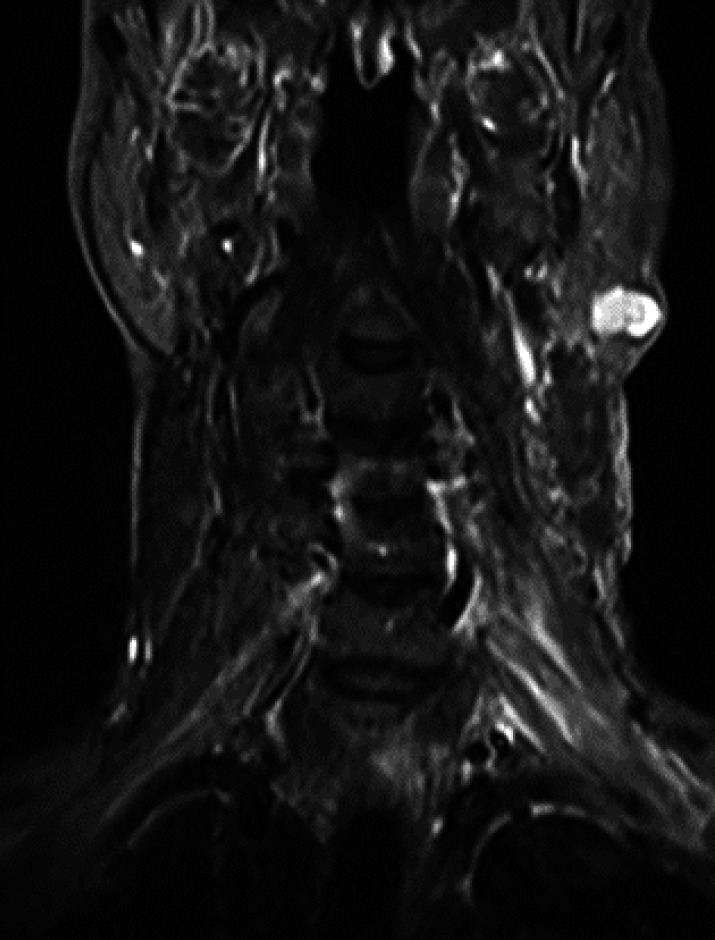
MRI showed multiloculated small cyst measuring 15x10 mm / small cyst measuring 15x10 mm in the Parotid gland
